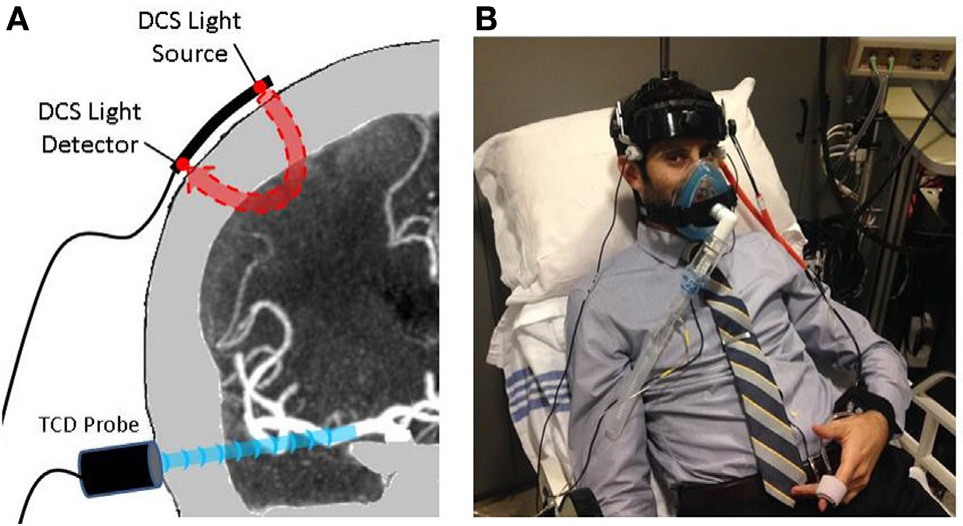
Protocol setup: (A) transcutaneous cortical cerebral blood flow monitoring by diffuse correlation spectroscopy (DCS) and middle cerebral artery trunk velocity monitoring by transcranial Doppler (TCD). (B) A subject prepared for the protocol with all cerebrovascular and cardiopulmonary equipment in place.
